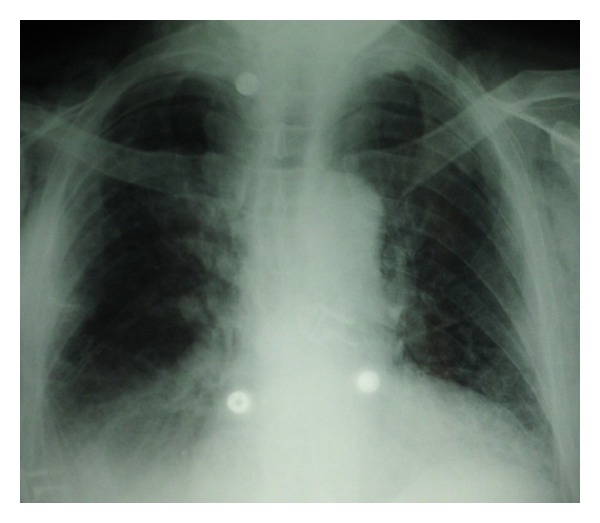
Postero anterior radiograph of the chest on Day 12.
